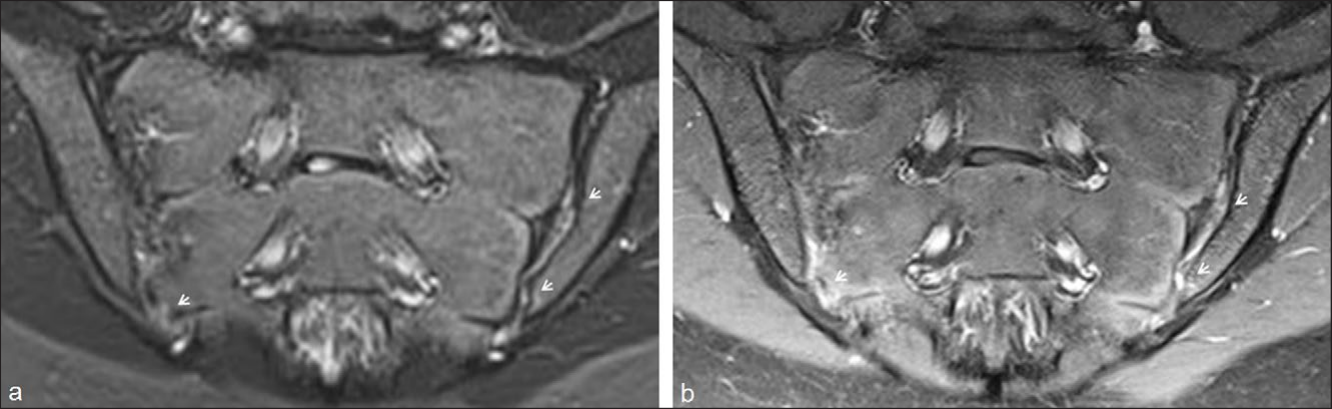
Synovitis representing active sacroiliitis in a 14-year-old girl with JSpA. a. Semicoronal STIR image shows high signal in the joint space of the left SI joint, discrete also in the caudal part of the right SI joint, with b. synovial enhancement representing synovitis in both SI joints on the contrast-enhanced fat-saturated T1-weighted image. No BME is seen.
